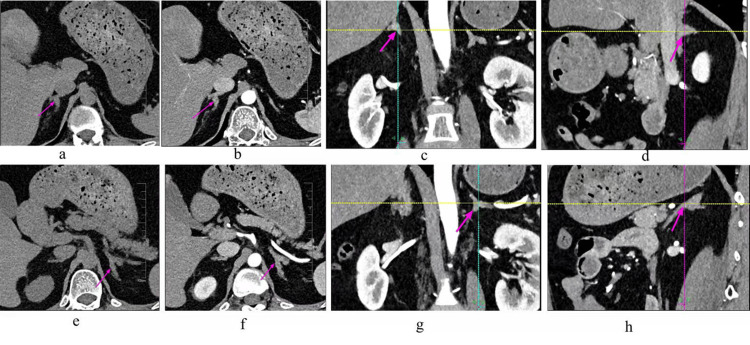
Bilateral adrenal CT scan with contrast enhancement (a) CT scan of the left adrenal gland.(b) Contrast-enhanced CT of the left adrenal gland. (c) Contrast-enhanced CT of the left adrenal gland in the coronal plane. (d) Sagittal CT with contrast of the left adrenal gland. (e) CT scan of the right adrenal gland. (f) Contrast-enhanced CT of the right adrenal gland. (g) Contrast-enhanced CT of the right adrenal gland in the coronal plane. (h) Sagittal CT with contrast of the right adrenal gland.
